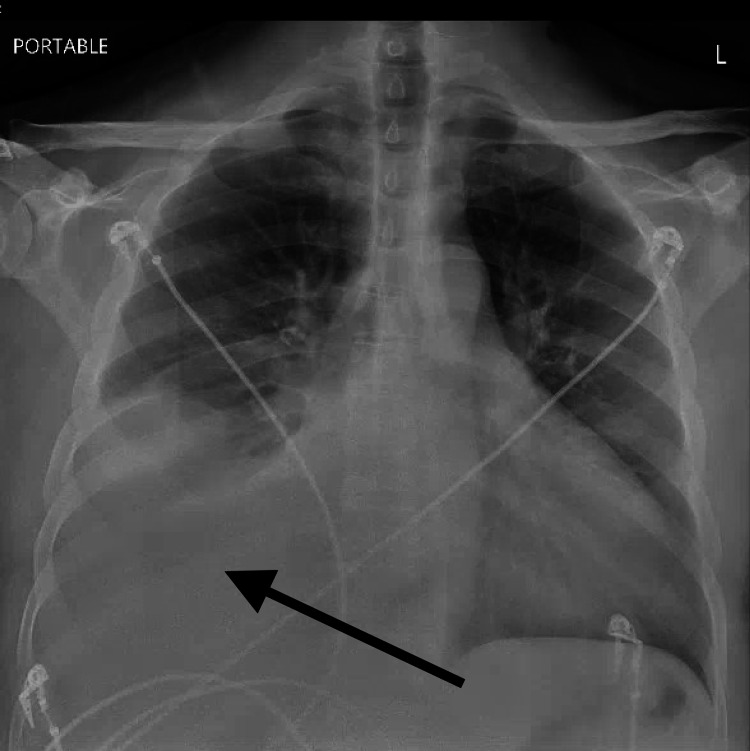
Chest X-Ray demonstrating right-sided pleural effusion Black arrow pointing to a pleural effusion
